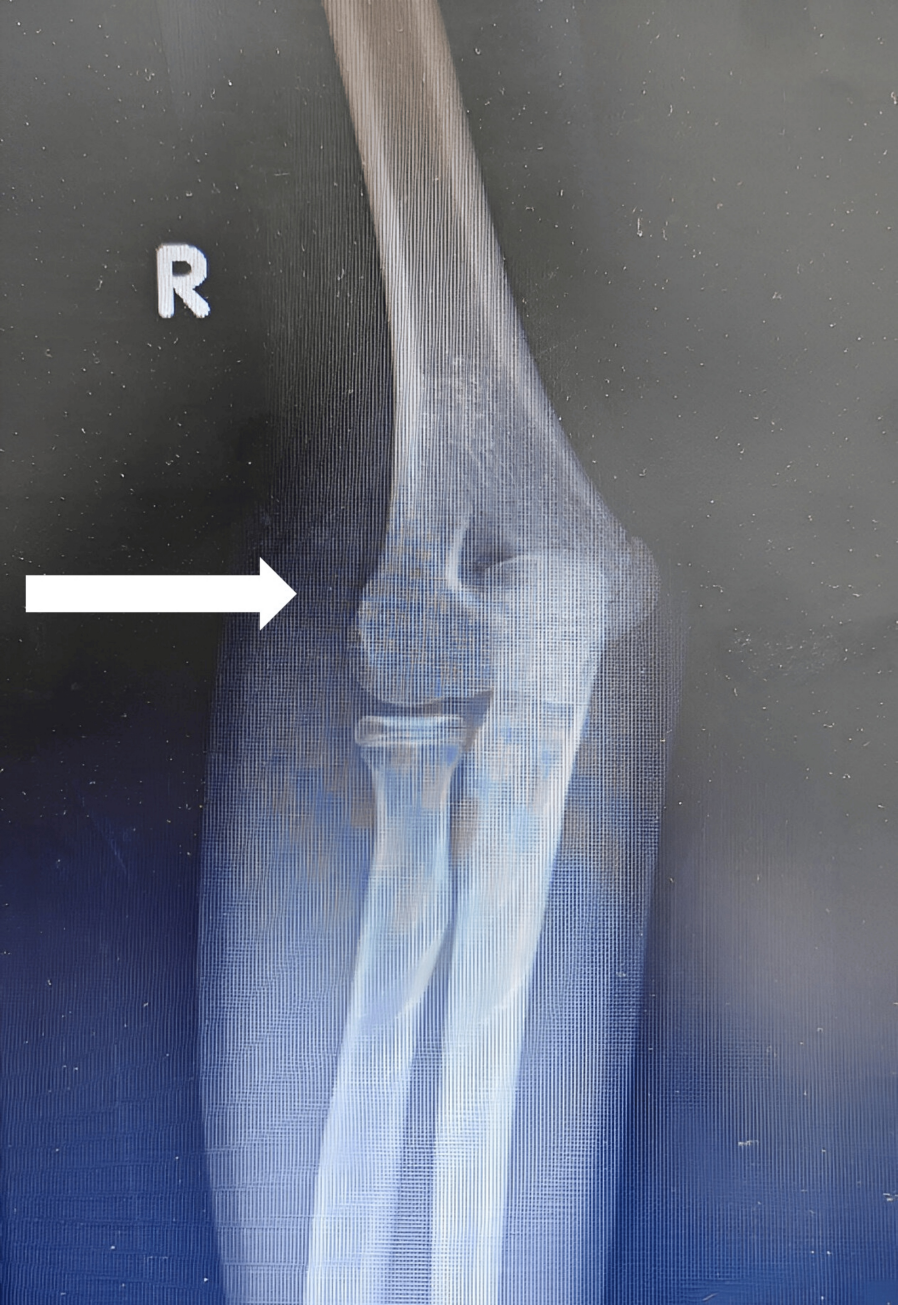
Post-resection AP X-ray right elbow shows complete resection (arrow).
